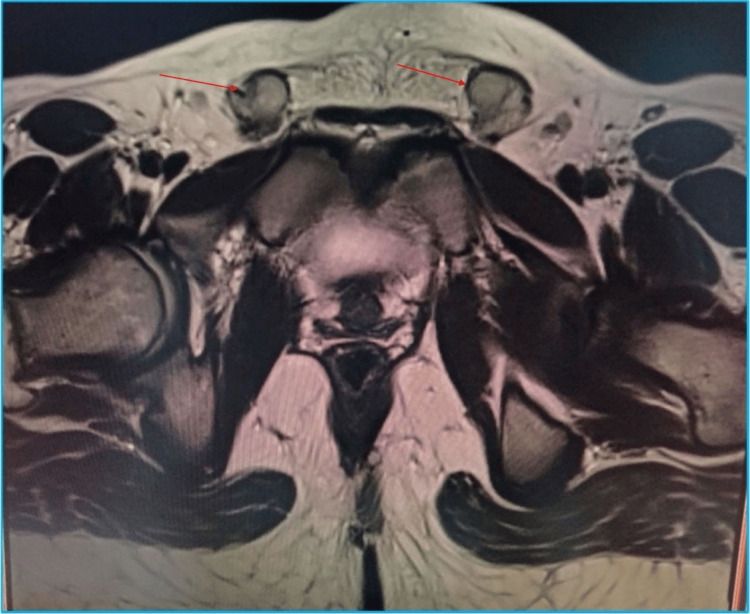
Abdominopelvic MRI. Axial MRI section showing bilateral inguinal gonadal tissue (red arrow). MRI, magnetic resonance imaging.
